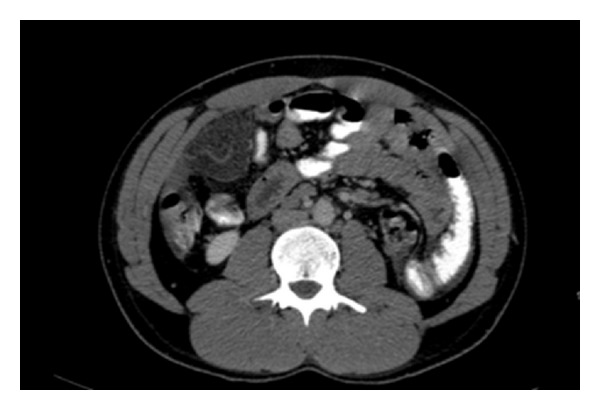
CT scan showing increased fat density mass in the upper quadrant.
